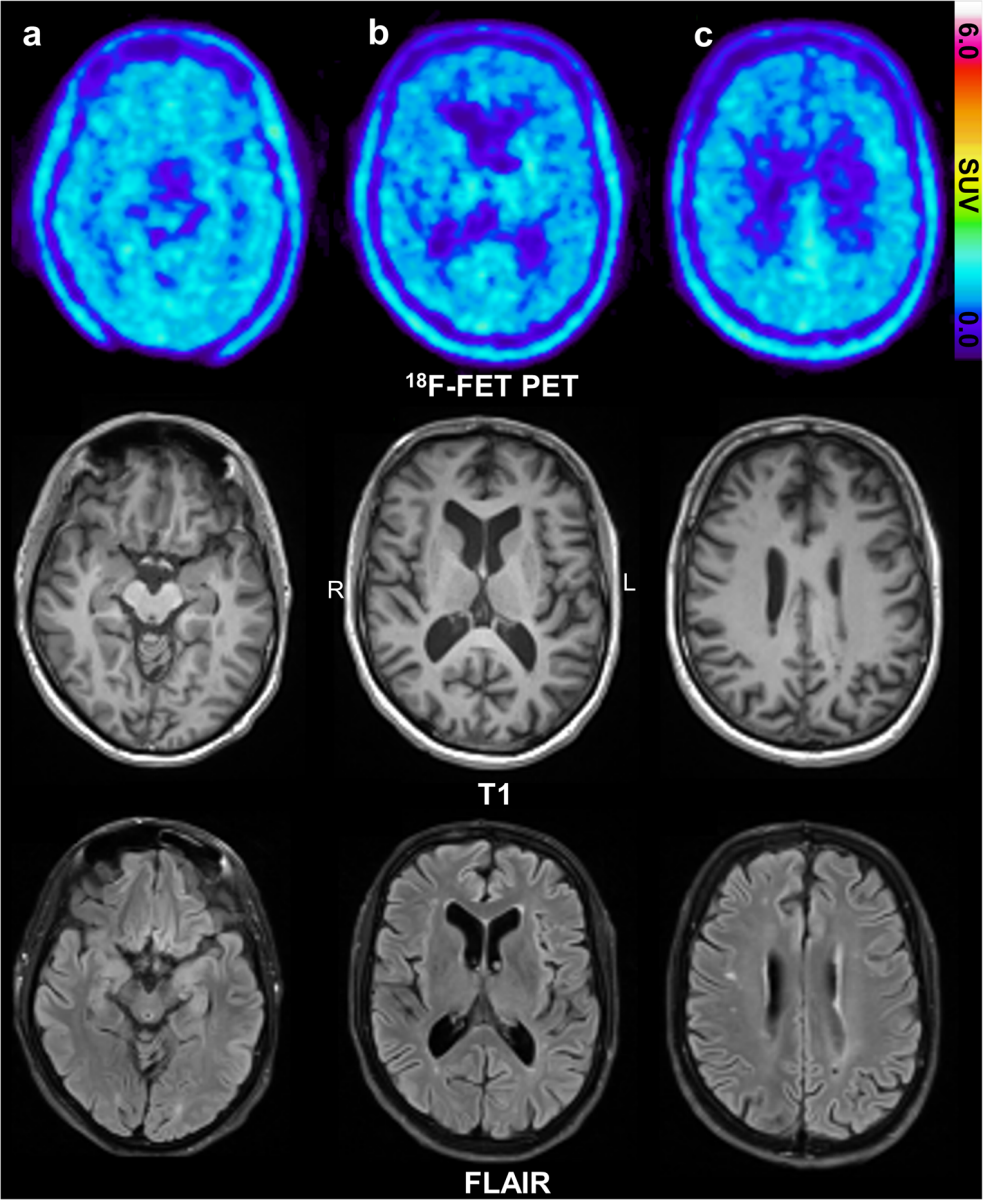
[^18^F]FET PET and MRI of patient no. 4 (Table 1) with bilateral temporal lobe epilepsy with nocturnal focal unaware and focal to bilateral tonic-clonic seizures. No abnormalities in [^18^F]FET uptake are visible. Representative horizontal sections at the level of the temporal lobe (a), the basal ganglia (b), or just below the centrum semiovale (c). Note a small subcortical lesion in the FLAIR sequence in the right hemisphere (c).
